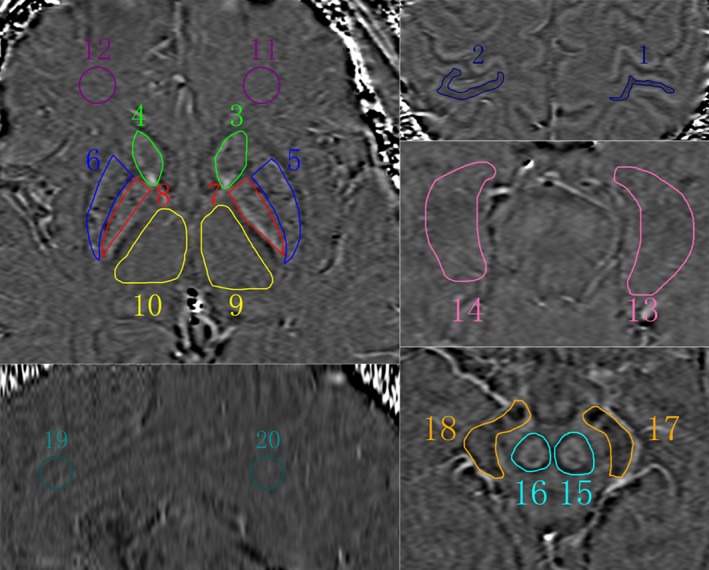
Region of interests in MR images. 1, 2: parietal cortex; 3, 4: caudate nucleus; 5, 6: putamen; 7, 8: globus pallidus; 9, 10: thalamus; 11, 12: frontal white matter; 13, 14: hippocampus; 15, 16: red nucleus; 17, 18: substantia nigra; 19: anterior cingulate cortex; 20: posterior cingulate
